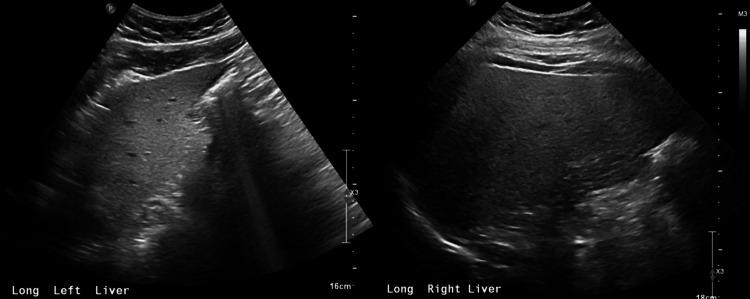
Abdominal ultrasound showing diffusely increased hepatic echogenicity No suspicious lesions were seen
